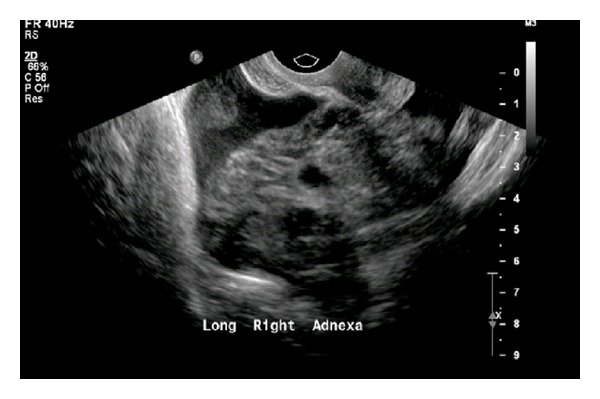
Pelvic ultrasound (long view, right adnexa) demonstrates a fluid collection of mixed echogenic and anechoic material surrounding the right ovary.
